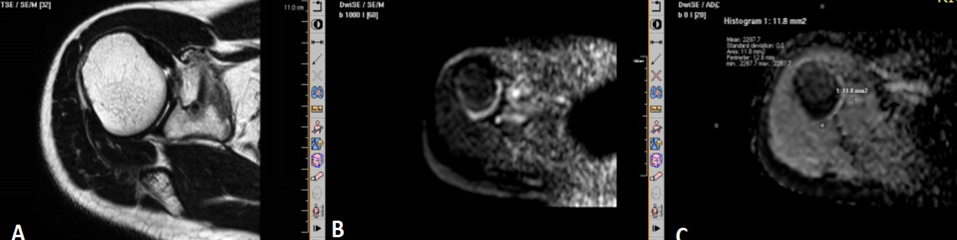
T_2_* weighted MR image (A) showing a small hypointense focal lesion in the right scapula just adjacent to the glenoid. The lesion on diffusion-weighted image (B) and ADC map (C) shows an ADC value of 2.2. This patient had classical clinical symptoms of Osteoid osteoma, CT scan (not shown) showing typical nidus and was treated for the same. ADC, apparent diffusion coefficient.
